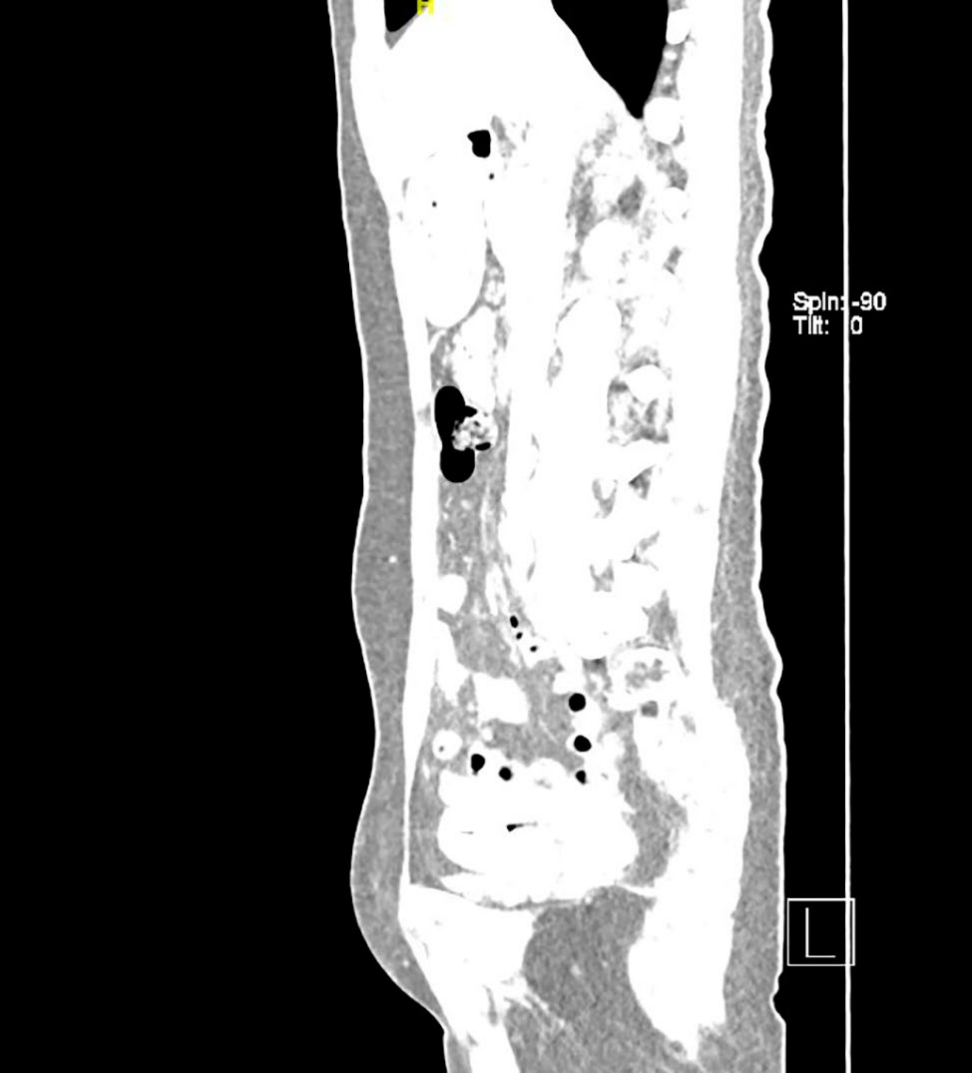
Postoperative thoraco-abdomin-pelvic CT showed no obvious residual filler
